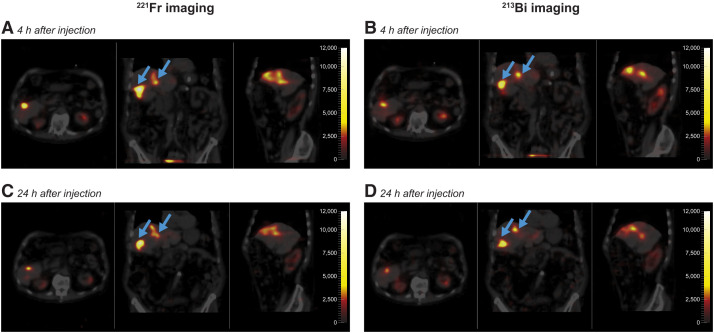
Example of quantitative reconstruction of ^221^Fr (A and C) and ^213^Bi (B and D) fused with CT images for representative patient (patient 3) at 4 (top) and 24 h postinjection (bottom). Arrows indicate tumor locations. SPECT images were acquired in 2 energy windows: 218.2 keV (±20%) to localize ^221^Fr and 440 keV (±20%) to localize ^213^Bi.
